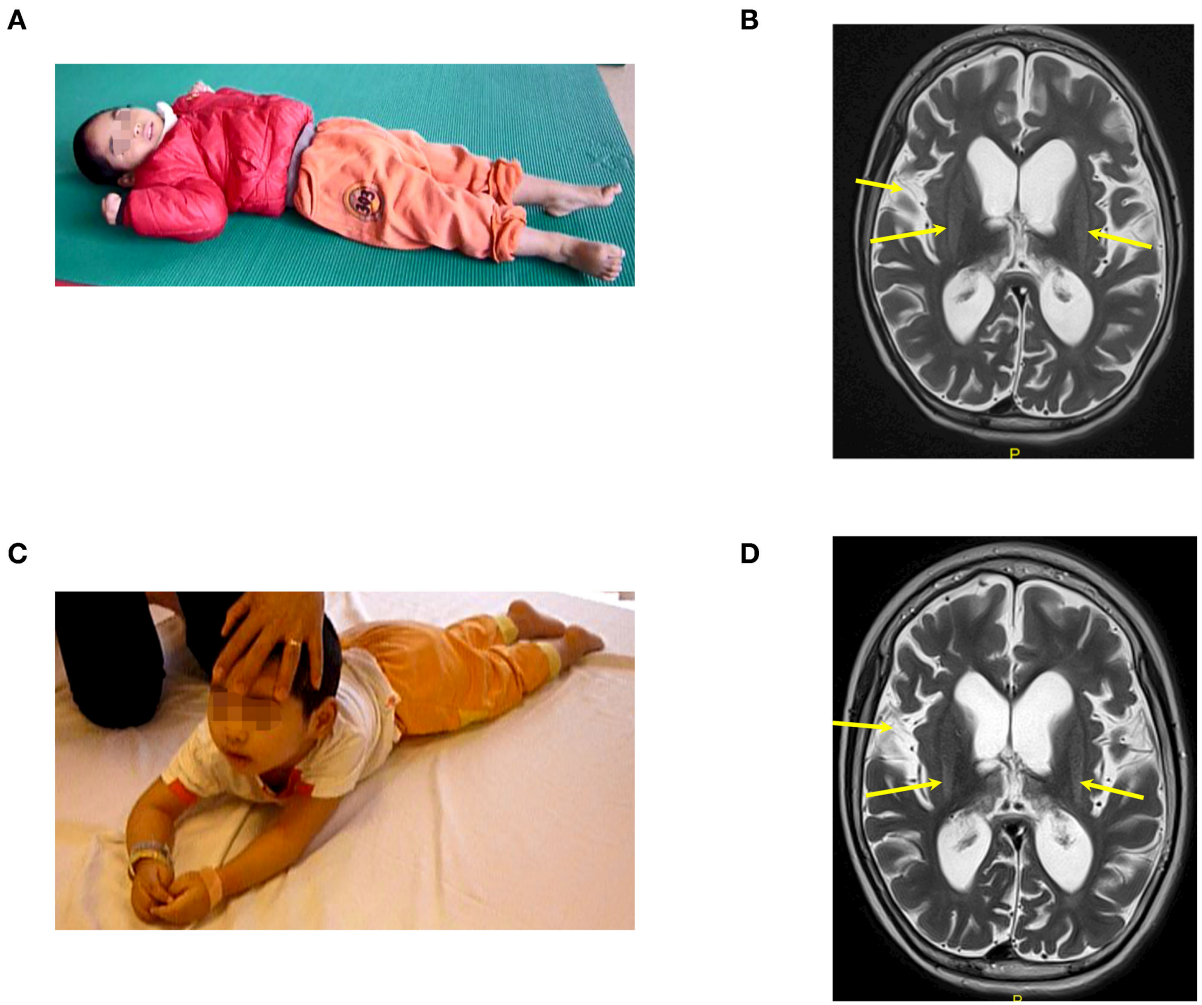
The patient (case 2) before and after autologous BMMNC transplantation. (A) Thirteen months after the drowning before BMMNC transplantation, the patient is in a VS without awareness, no motor function, and exhibited muscle spasticity at level V. (B) Diffuse cerebral atrophy at supratentorial region and abnormal signal at bilateral putamen on brain MRI before transplantation (arrows). (C) Six months after the fourth transplantation, the patient could respond to sound and music, smile or cry during communication, and turn and lift his head up when lying prone. (D) However, there was no significant change in brain MRI after transplantations in comparison with that before transplantation.
